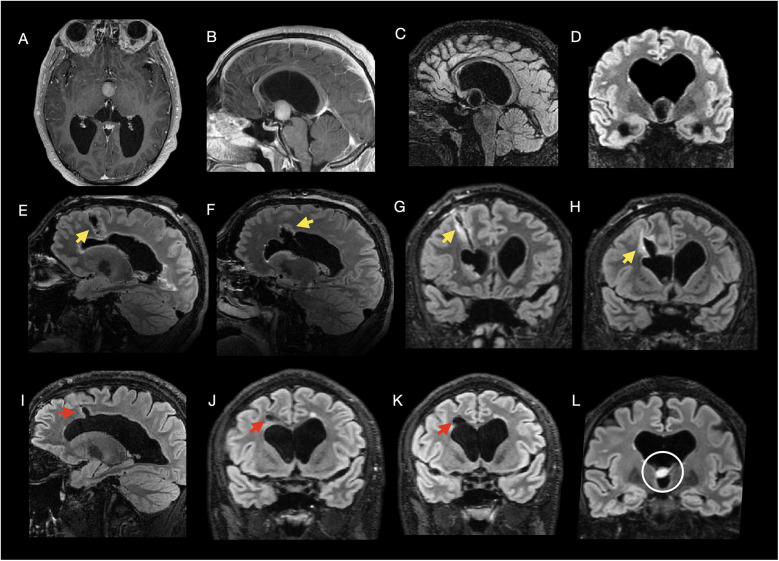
Multimodal imaging of a 22-year-old female with chronic headache due to a third ventricular colloid cyst, treated via open interhemispheric transcallosal resection. Preoperative images (A–C): Axial T2-weighted (A), sagittal post-contrast T1-weighted (B), and coronal 3D T2-weighted FLAIR (C) images demonstrate a smoothly contoured, non-enhancing hyperintense lesion measuring approximately 9 mm in diameter in the anterior third ventricle at the foramen of Monro, consistent with a colloid cyst, without ventricular enlargement. Postoperative day 1 MRI (D–F): Sagittal (D) and coronal (E,F) 3D T2-weighted FLAIR images show expected postoperative changes without definite residual enhancement, and focal hyperintensity around the corpus callosotomy tract and surgical corridor, without surrounding edema. FouR,Month folloW,Up MRI (G–I): Sagittal (G) and coronal (H,I) 3D T2-weighted FLAIR images show no residual lesion or recurrence and demonstrate the surgical callosotomy without surrounding hyperintensity or parenchymal tissue loss. Arrow key: Yellow arrows: postoperative hyperintensity (D,F); red arrow: callosotomy defect (G).
